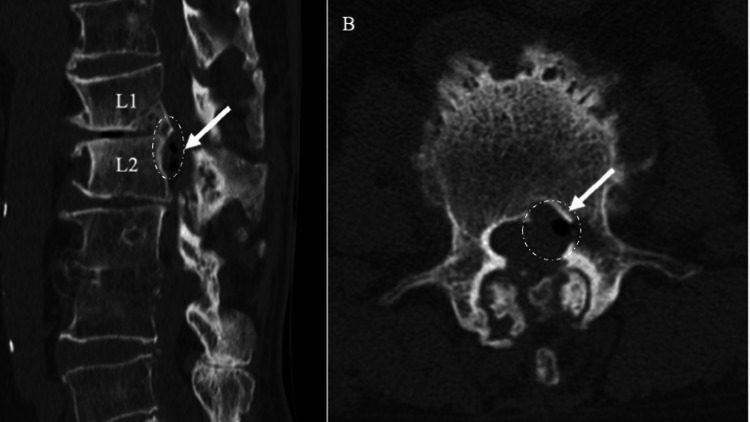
Immediate Postoperative CT Scan After FED-TFA Sagittal (A) and axial (B) CT images obtained on postoperative day 1 demonstrate a marked reduction of the epidural gas component *(*arrow and *dotted circle)*. FED-TFA: full-endoscopic discectomy via the transforaminal approach.
